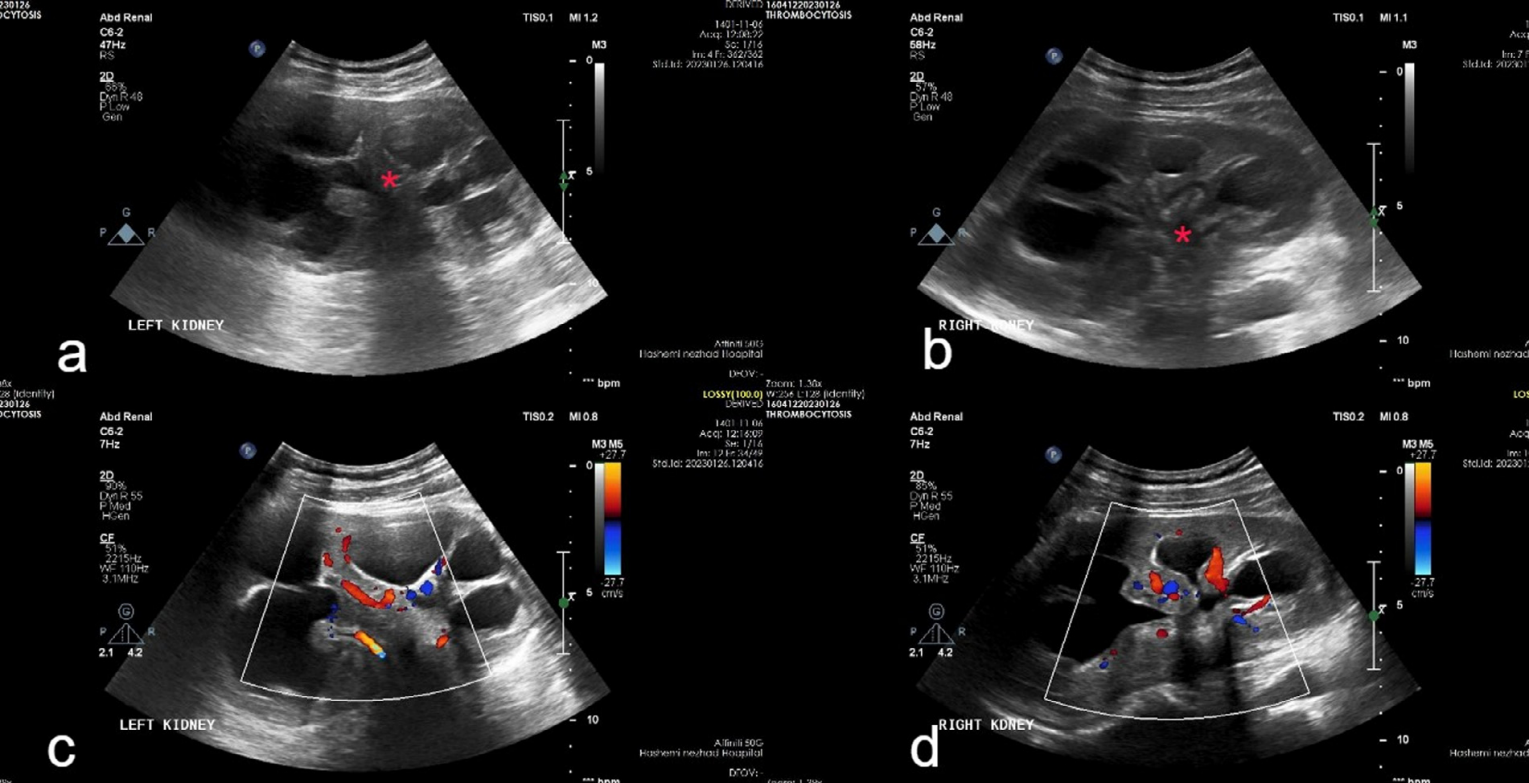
Urinary ultrasonographic examination of the left kidney shows dilated calyxes with echogenic fibrotic tissue in the renal pelvis. (B, D) Also, the same findings are seen in the right kidney.
